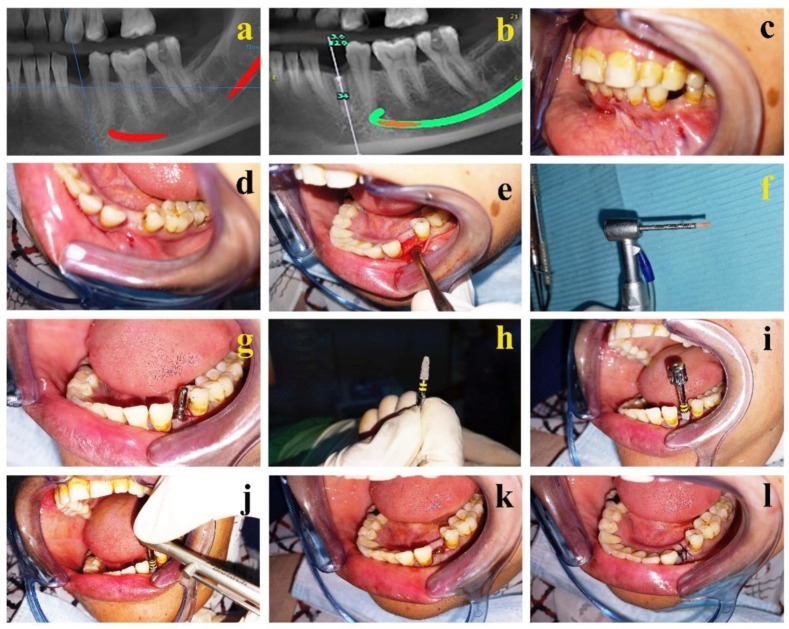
Surgical re-entry at 3 months for bone core biopsy and implant placement: (a) pre-operative CBCT of the extraction site at 3 months showing complete healing of the extraction socket; (b) pre-operative CBCT of the virtual implant placed at the extraction site; (c) pre-operative clinical presentation of the inter-occlusal space in the centric occlusion before the implant placement; (d) intra-oral picture showing complete mucosal healing after extraction of the first premolar before the implant placement; (e) reflection of a full mucoperiosteal flap; (f) bone core biopsy obtained harvested from the augmented area by the trephine bur before implant site preparation; (g) parallel pin in place showing the parallelism of the implant to the neighboring teeth; (h) macrostructure of the NeoBiotech tapered resorbable blast media (RBM) dental implant (3.5 × 11.5 mm); (i) implant inserted into the osteotomy site; (j) complete insertion of the implant in the osteotomy site using manual torque wrench; (k) cover screw placed over the dental implant before closure of the flap; and (l) mucoperiosteal flap repositioned and sutured.
